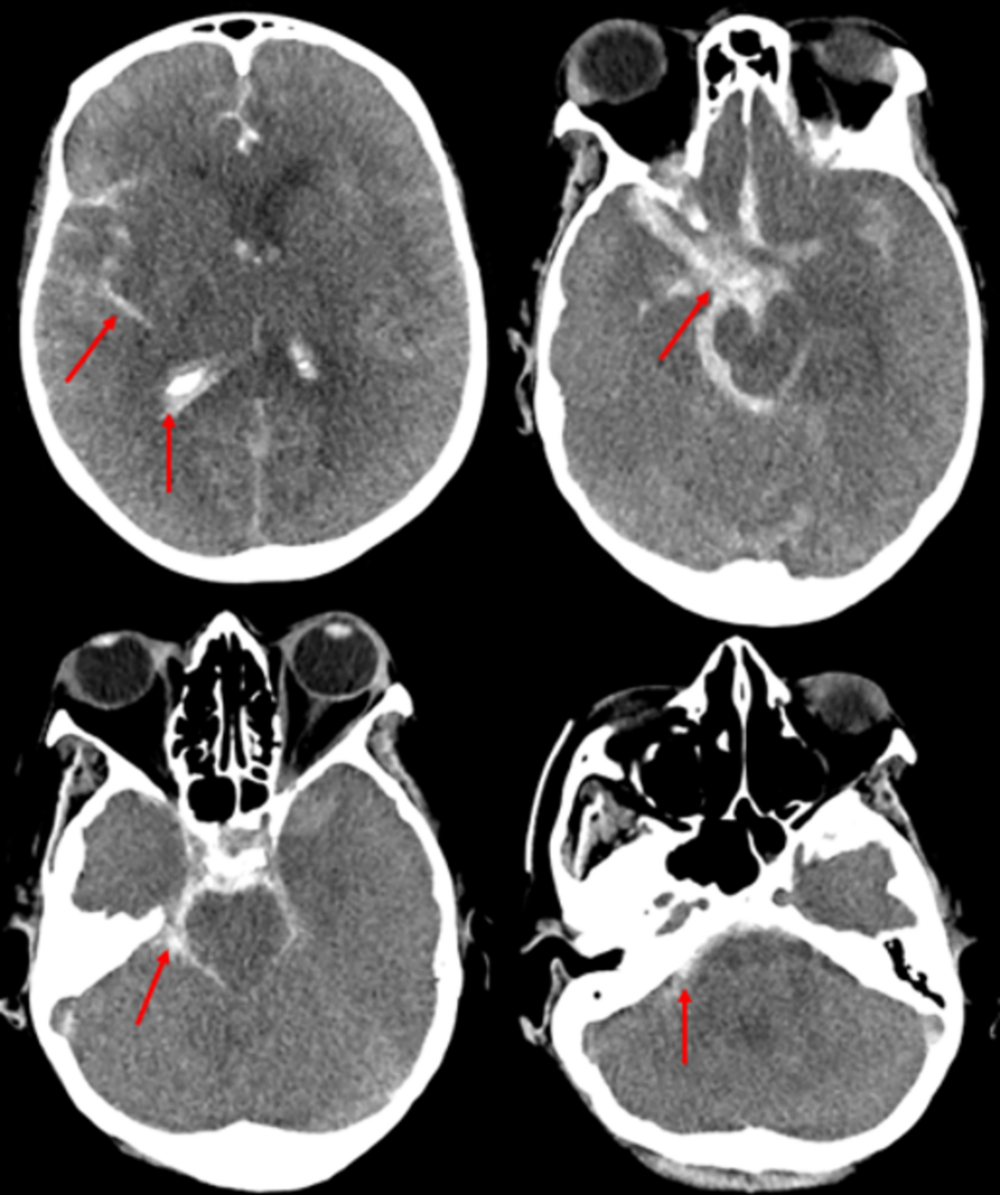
Axial CT head without intravenous contrast There is a large subarachnoid hemorrhage extending into the sulci, ventricles, and basal cisterns (red arrow). There is diffuse cerebral edema with effacement of the sulci and loss of the gray-white differentiation CT: computed tomography
